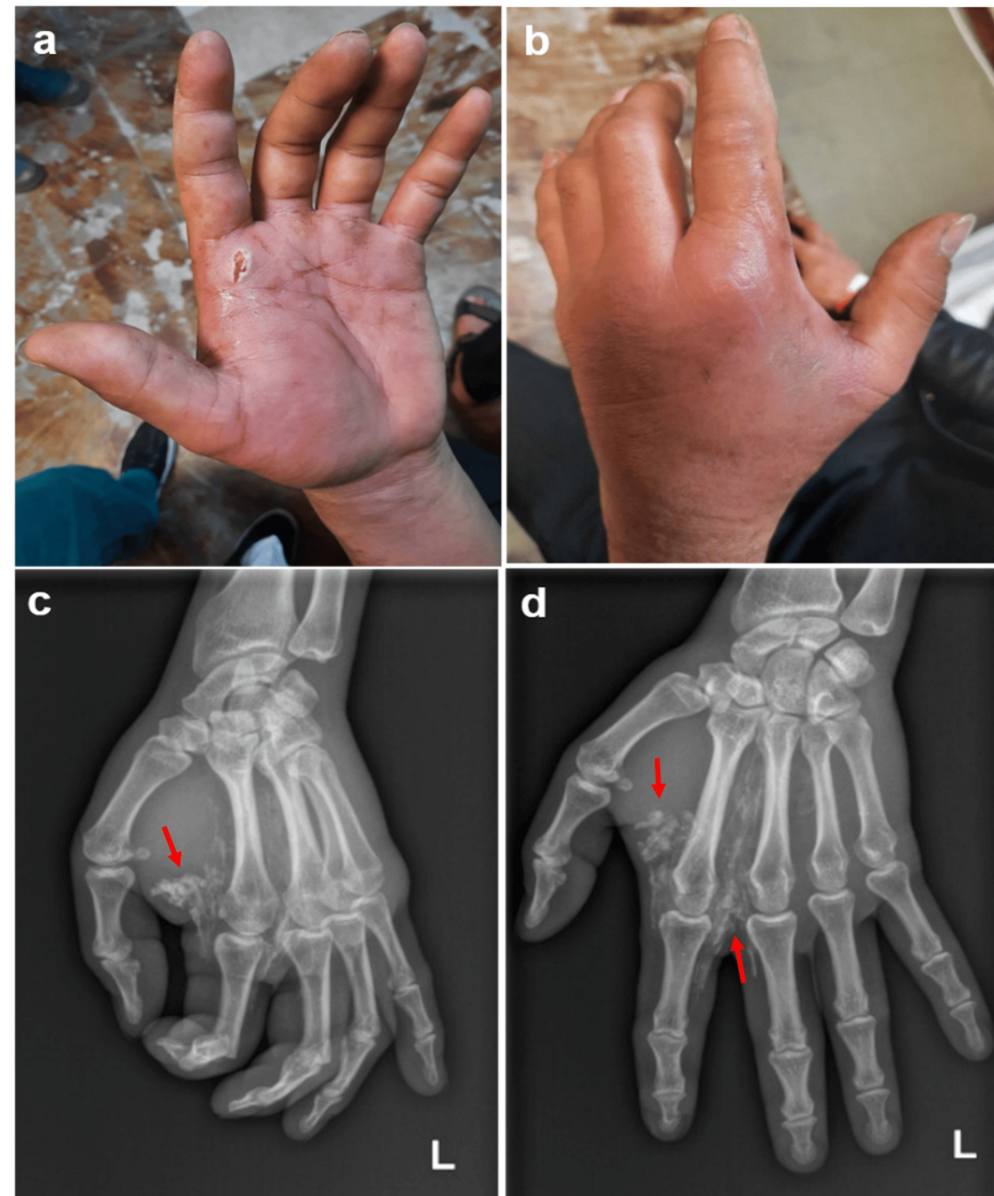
Case 3 (a, b) Clinical presentation with a small punctiform wound at the injection site, located at the palmar metacarpophalangeal level of the index finger. (c, d) Admission X-ray of the left hand showing extensive infiltration of dense material (arrows) in the thenar and mid-palmar regions, extending into the second and third fingers.
